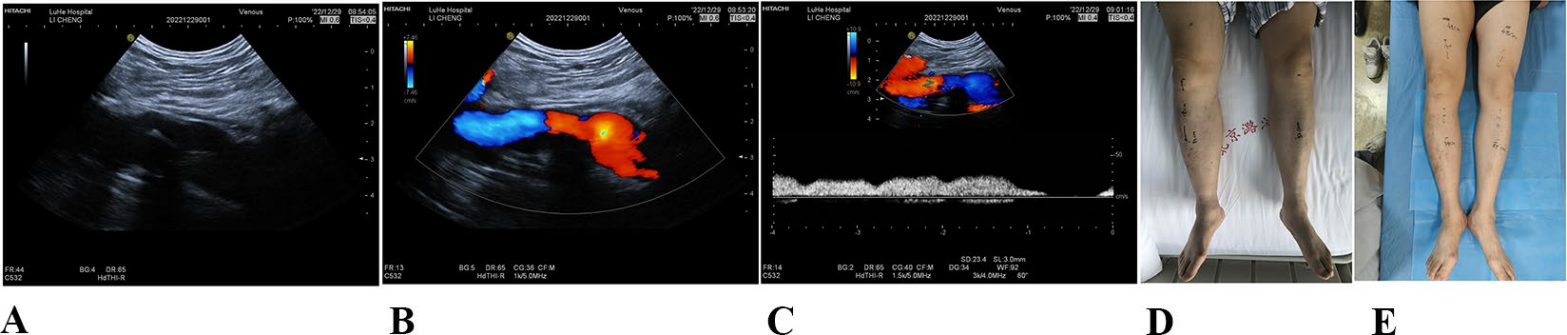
Ultrasound at 3 months after surgery (A) The long solid arrow shows the SFV, and the long arrow shows the AV. The short solid arrow shows the ring, and the cross mark shows the ligation point of the SFV. (B) The diverted axillary vein is unobstructed; the arrow shows the healthy axillary vein valve. (C) During the valsalva maneuver, no blood reflux was recorded in the diverted vein valve. (D and E) The patients lower limbs before and at 3 months after surgery, which showed slight edema in the right calf, but the leg circumference at 15 cm below the knee was 1 cm less than that before surgery
